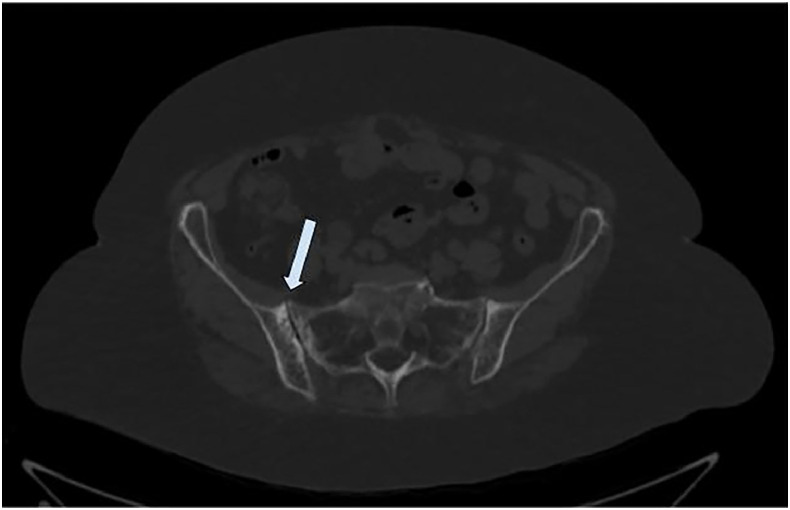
Degenerative changes in the right sacroiliac joint. Axial CT image of bone window through the pelvis shows degenerative changes in the form of lytic and sclerotic changes in the right sacroiliac joint (white arrow).
